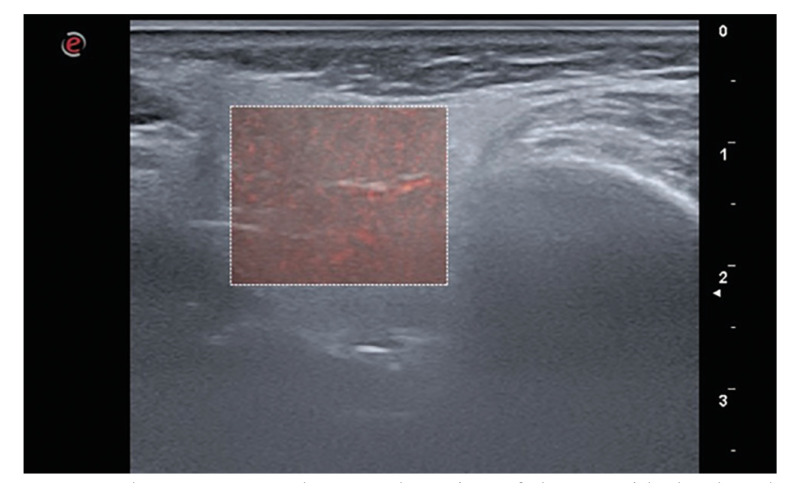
The transverse ultrasound section of the parotid gland, red coloured areas indicating the presence of microvessels and associated perfusion.
